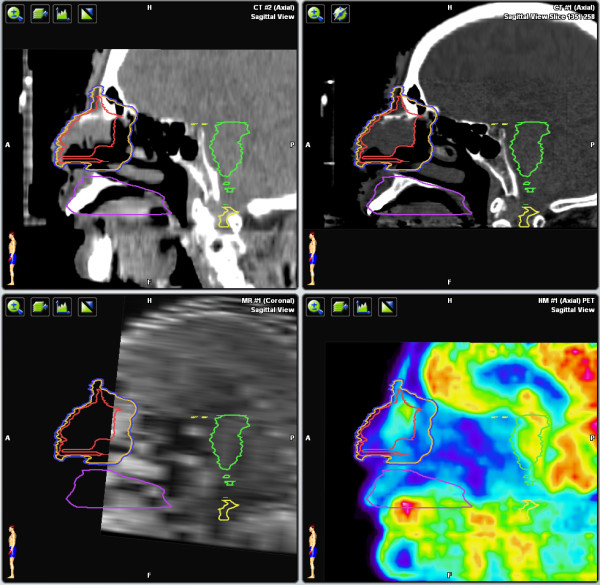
CT, MRI, and PET sagittal views. Patient was unable to tolerate the MRI, resulting in motion artefact.
